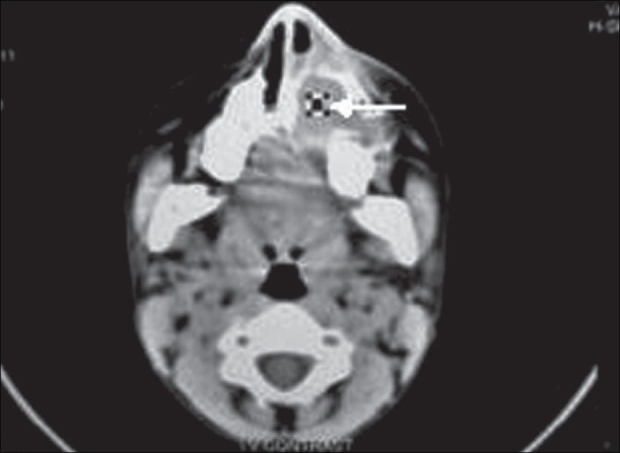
Axial CT scan of the orbits and sinuses showing the cyst bulging into the nasal cavity and the tooth in the left maxillary antrum (white arrow)
